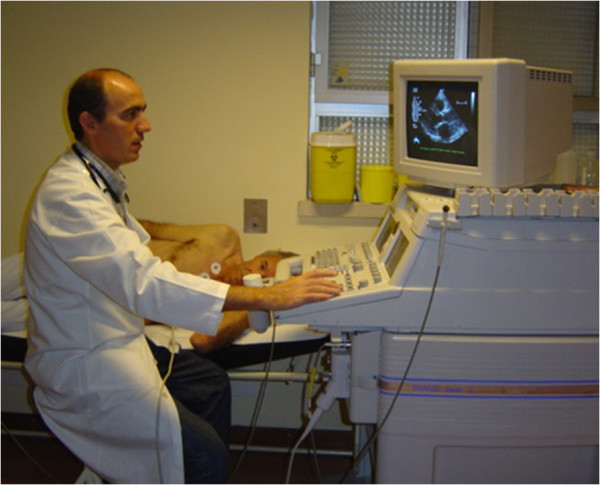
Echocardiographic data acquisition with the patient in left lateral decubitus before exercise.
